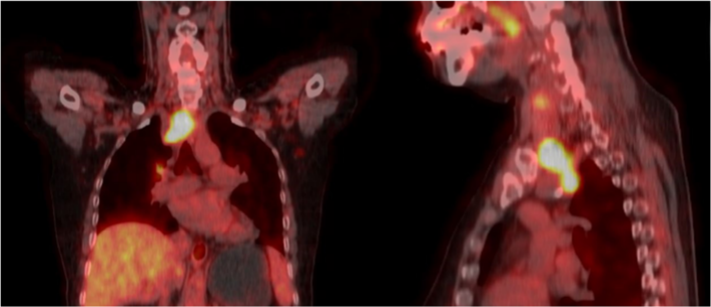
PET-CT revealed a high metabolic tumor under sternal bone
